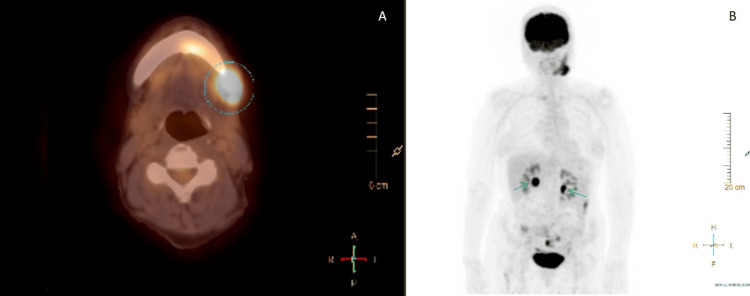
PET-CT scan demonstrating systemic involvement consistent with Ann Arbor stage IV follicular lymphoma. (A) Axial PET-CT image showing increased fluorodeoxyglucose (FDG) uptake in the left buccal space and mandibular body (blue circle), corresponding to the primary mandibular lesion, with a maximum standardized uptake value (SUVmax) of 10.5 — suggestive of metabolically active lymphoproliferative disease. (B) Whole-body maximum intensity projection (MIP) reveals additional FDG-avid sites, including low-grade diffuse uptake in the sternum and right proximal femur. Multiple foci of low-to-moderate FDG uptake are also observed in the gastrointestinal tract, particularly in the transverse colon (green arrows), consistent with multifocal extranodal involvement. These findings supported the diagnosis of disseminated disease and were crucial for staging.
